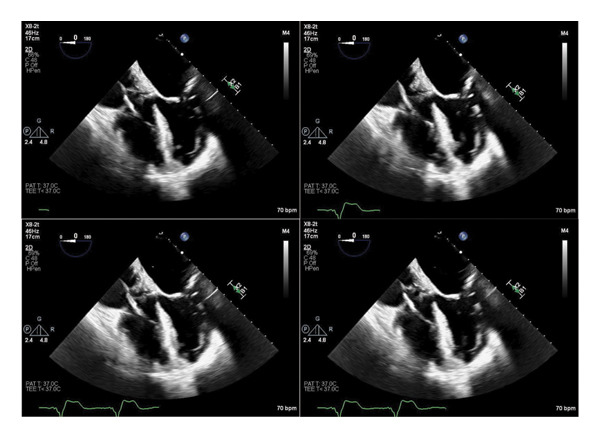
Transesophageal echocardiogram (TEE), midesophageal four‐chamber view, demonstrating no evidence of valvular vegetations or pacemaker lead–associated vegetations.
